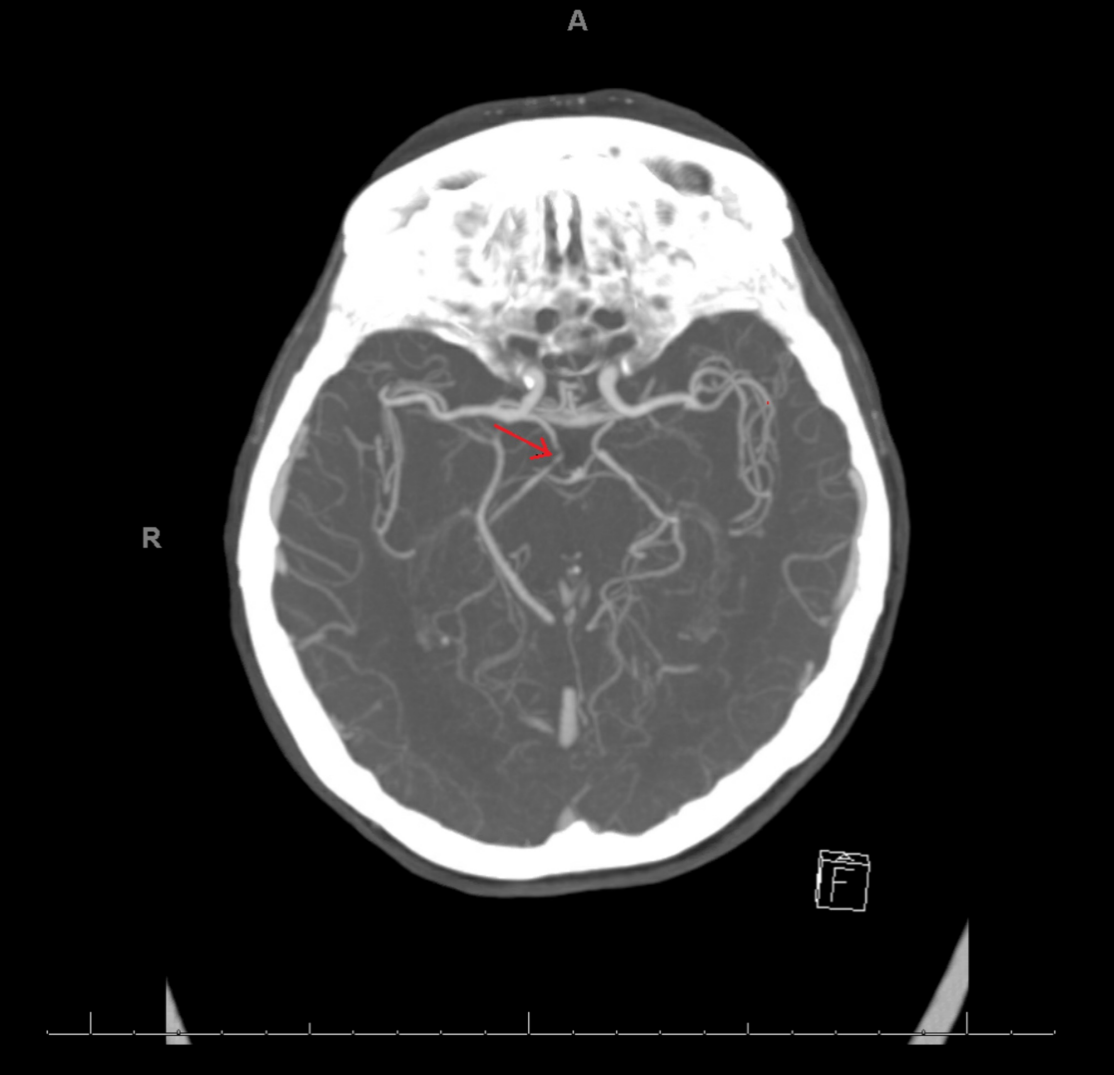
CTA of the head shows a mild to moderate short segment narrowing of the distal right P-comm artery, multi-focal narrowing of the right posterior cerebral artery. CTA, CT angiography
